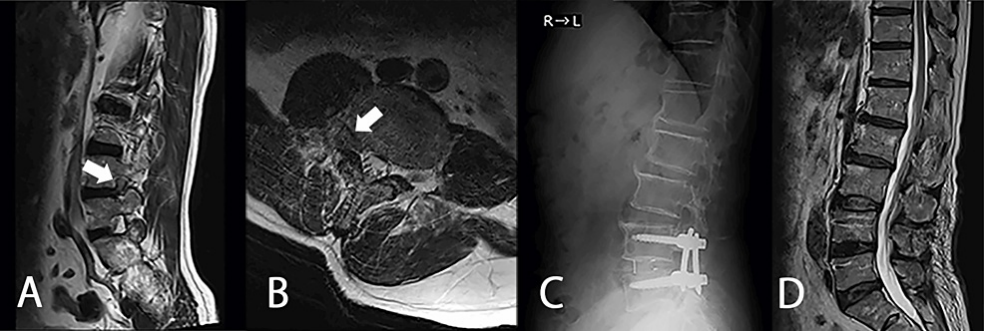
Initial images of case 2 A: T2WI sagittal section; B: T2WI axial section. Initial MRI scans of case 2 showed a herniated disc in RT. L4/5 intervertebral foramen (white arrows). C: Lateral lumbar X-ray, D: T2WI sagittal section. Images after the TLIF operation MRI: magnetic resonance imaging; TLIF: transforaminal lumbar interbody fusion
